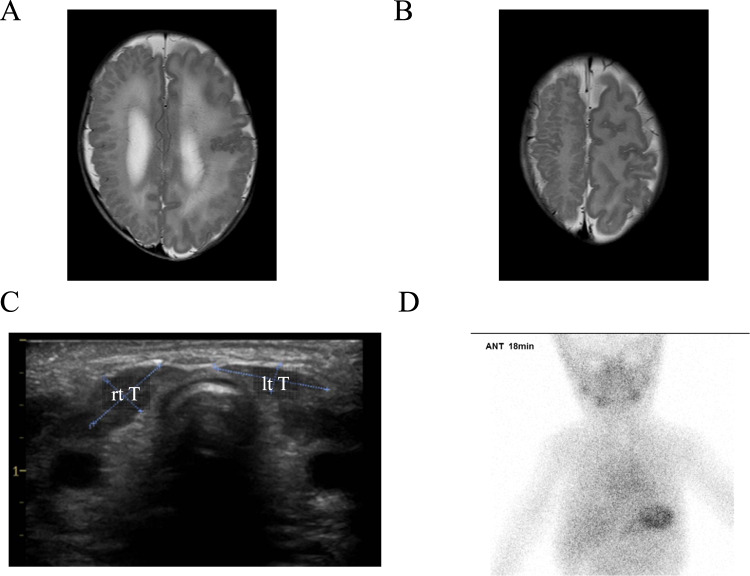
Images of the patient. A, B MRI of the brain showed asymmetrical megalencephaly with bilateral polymicrogyria. C Thyroid ultrasound examination revealed that both the right and left thyroid lobes were small. D Thyroid scintigraphy by ^99m^Tc pertechnetate showed no functional thyroid gland.
